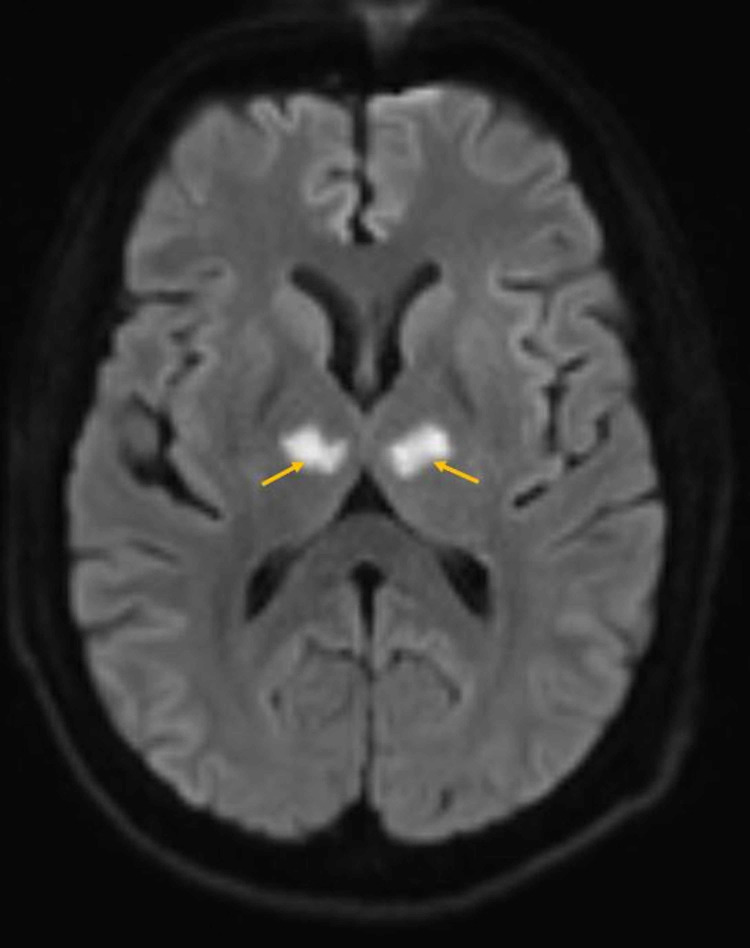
Brain MR diffusion weighted (DWI) axial image. Bilateral anterior thalamic high signal intensity is seen.
